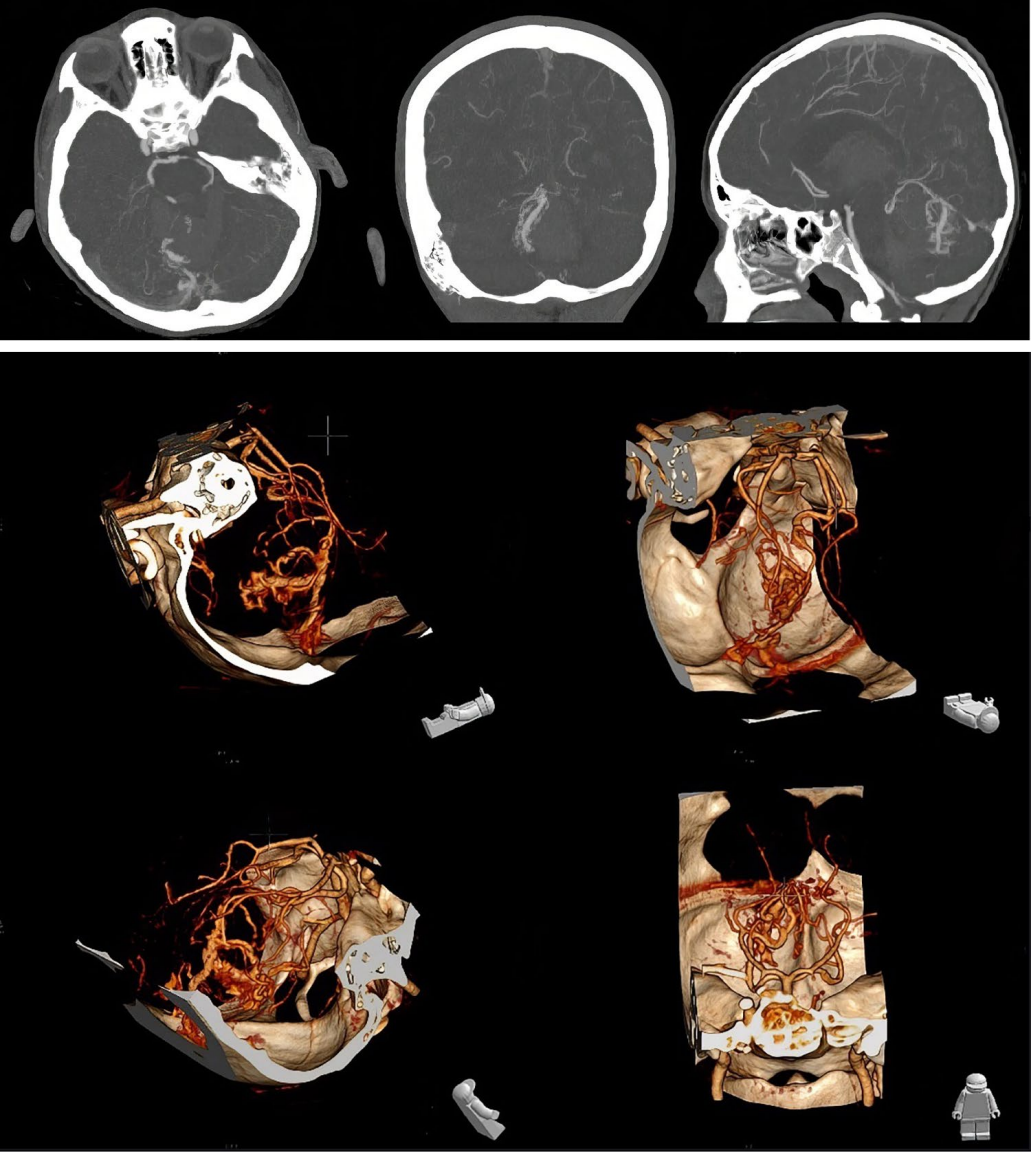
CT angiography with 3D reconstruction of the AVM
